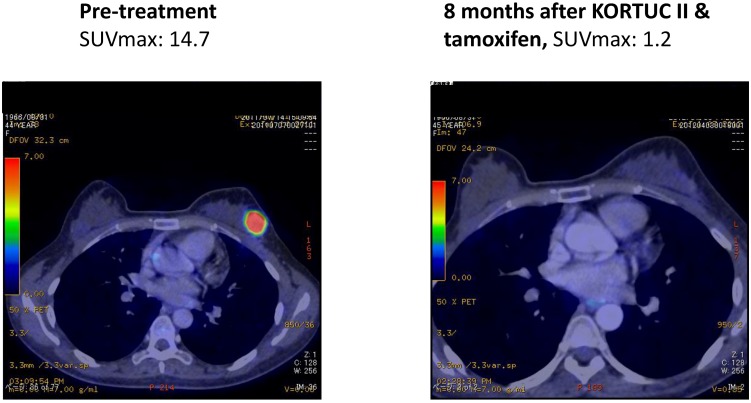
Case 44. 44 years old female patient with left breast cancer (cT2N0M0, ER: +, PgR: +, HER-2: 1+, Ki-67 index: 13%) treated with radiosensitization (KORTUC II) and without systemic chemotherapy. Left: PET-CT image at pre-treatment. Tumor diameter is 35 mm and SUV-max value is 14.7. Right: PET-CT image at 8 months following KORTUC II. Tumor is not recognized and SUV-max value on the region is approximately 1.2.
